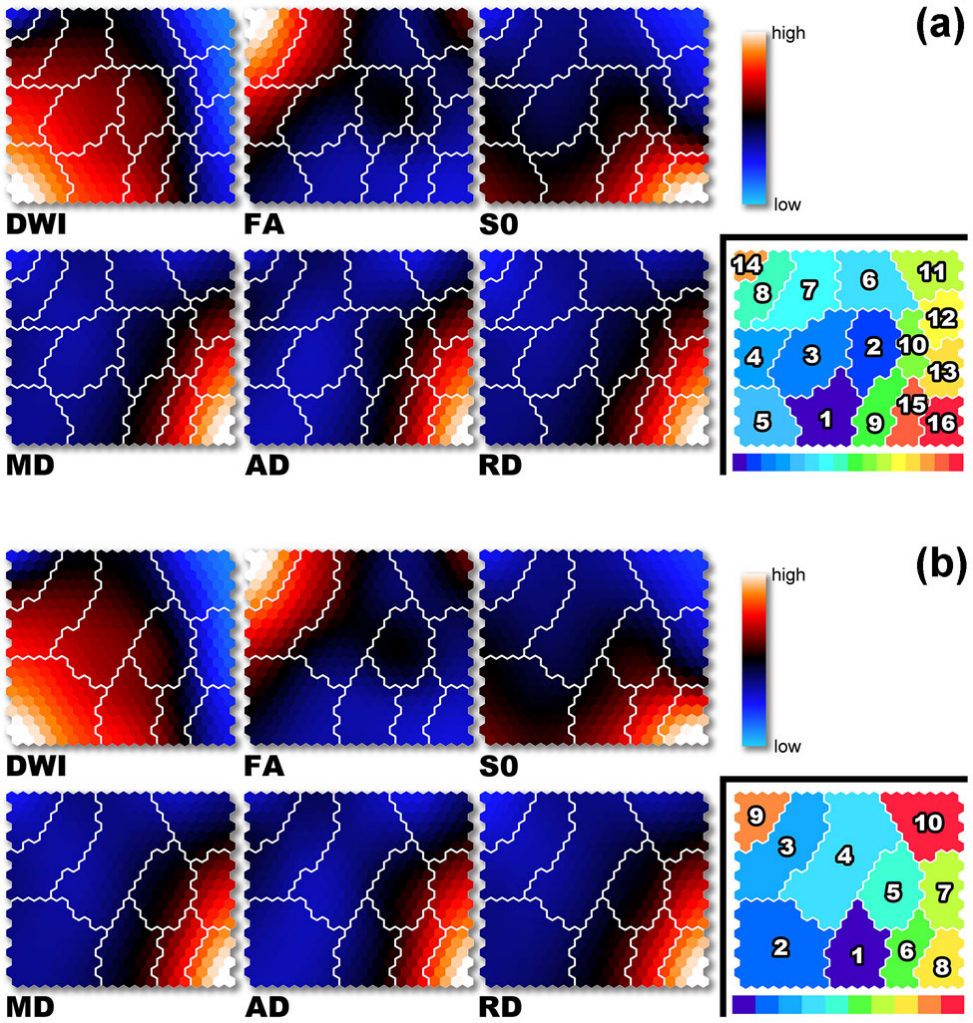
Visualization of six DTI‐based variables on component planes with 20 × 20 SOM. Each node (protocluster) is colorized from blue to red according to the intensities in each diffusion tensor image. The white lines between nodes denote interclass borderlines obtained by KM++ with *K* = 16 (a) and *K* = 10 (b) on SOM. SOM component planes can help to interpret detailed intensity profiles or patterns in each diffusion tensor image. Each class number corresponds to the intensity on DTI‐based clustered images. DTI, diffusion tensor MRI; SOM, self‐organizing map; DWI, diffusion‐weighted imaging; FA, fractional anisotropy; S0, raw T2 signal without diffusion weighting; MD, mean diffusivity; AD, axial diffusivity; RD, radial diffusivity.
